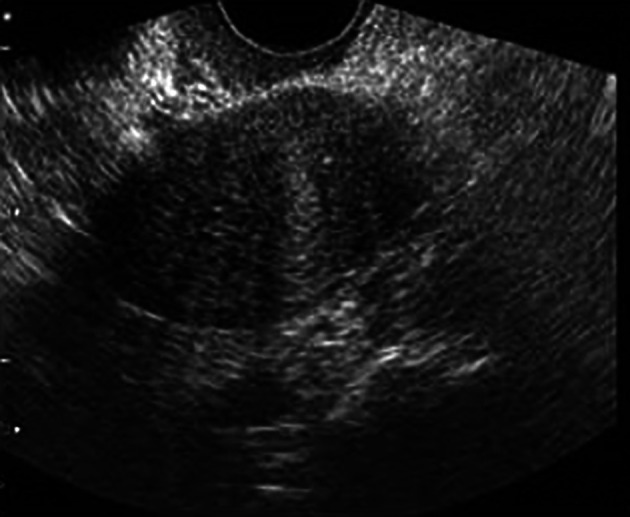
Ultrasound of the abdomen and pelvis showing ovarian cyst and ascites.
